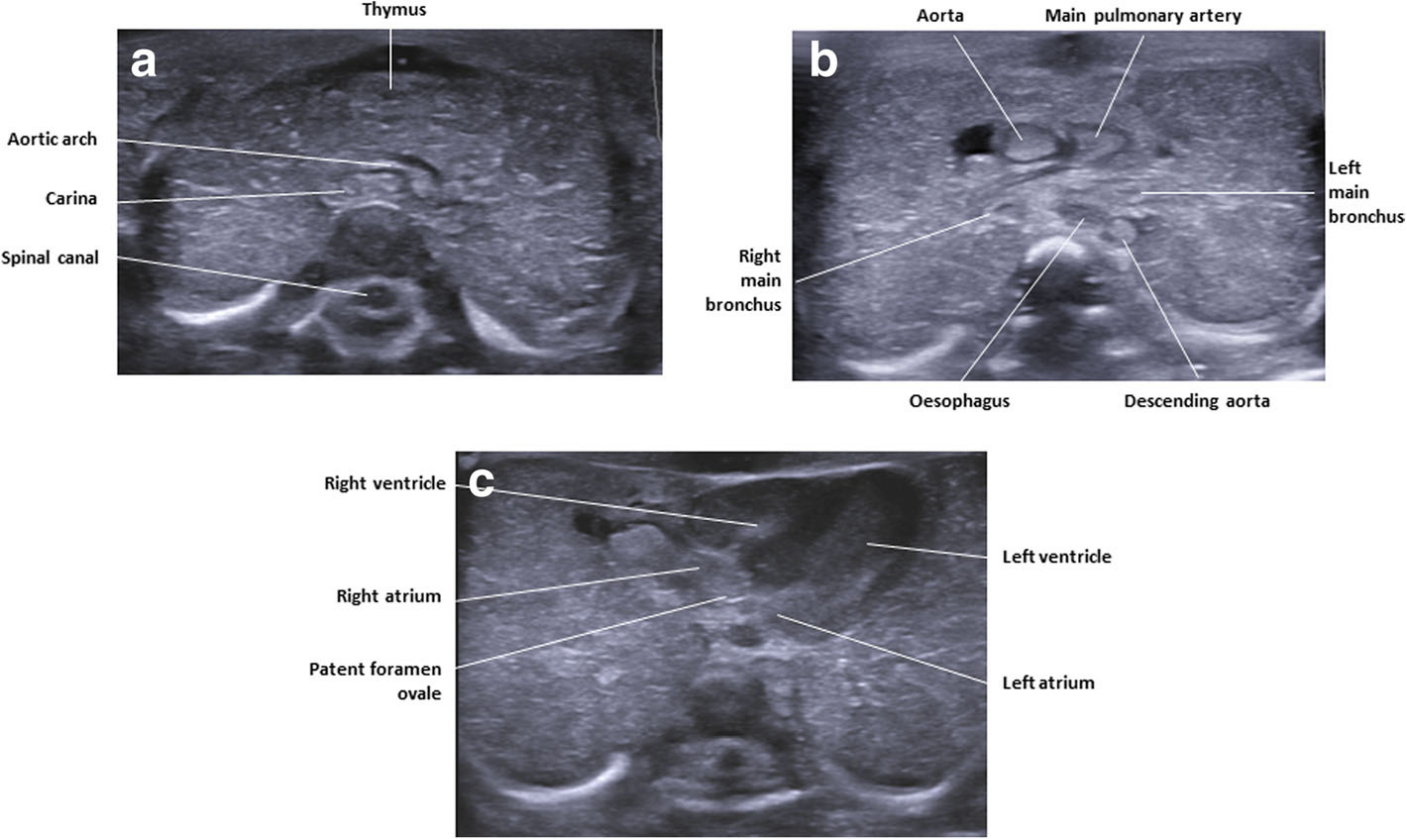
Labelled transverse postmortem ultrasound images of the chest in a 20-week gestational-aged foetus, obtained 4 days after death demonstrating normal anatomy. The labelled images are taken at level of the aortic arch (a), level of the main outflow tracts (b) and at the biventricular level of the heart (c). They serve to demonstrate the detail that can be gained with ultrasound imaging in a well-preserved foetus
